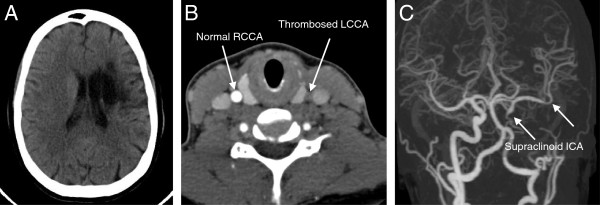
Imaging from 6 months following initial acute CVA. Brain CT showing encephalomalacia related to the previous infarct of the left basal ganglia and left insular cortex (left MCA territory). (A) Transverse slice through neck showing complete occlusion of left common carotid artery (B). Subtracted 3D CT angiogram showing a complete lack of flow in left internal carotid artery as well as a recanalization of the previous area of left MCA thrombosis (C).
